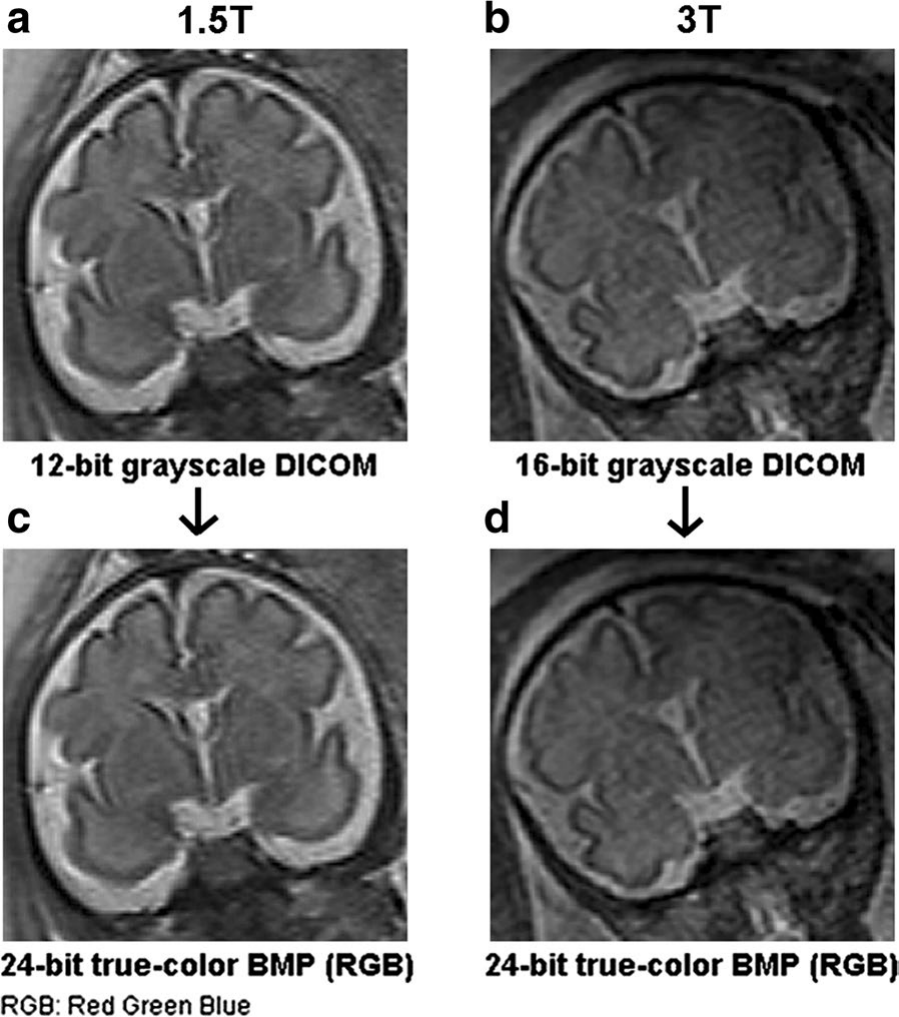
a 1.5 T MR image of a fetal brain: 12-bit DICOM format, coronal section. b 3 T MR image of a different subject: 16-bit DICOM, coronal plane. c Same image shown in “a” after conversion to BMP. d Same image shown in “b” after conversion to BMP
